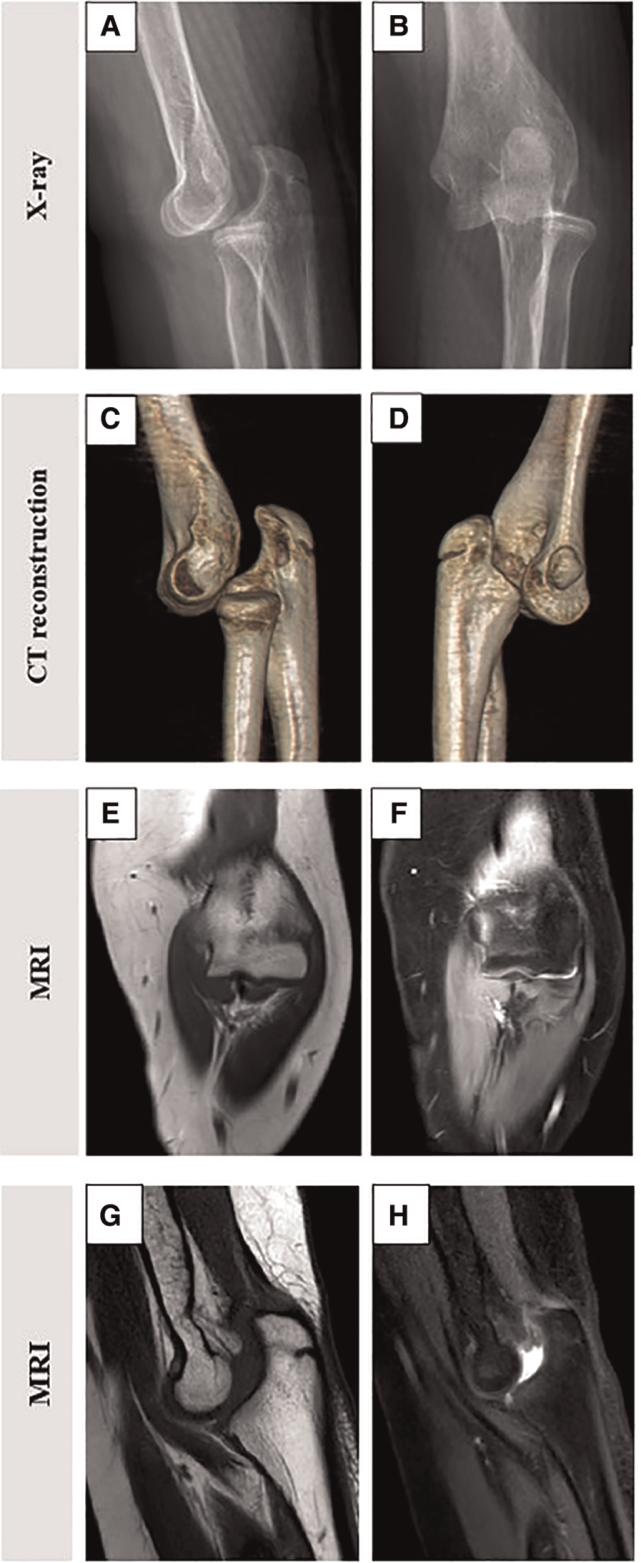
Preoperative imaging showing posterolateral dislocation of the left elbow. (A,B) Radiographs show anteriorly inferior slippage of the distal humerus without fracture; (C,D) Three-dimensional computed tomography shows a transverse defect of the coronoid process of the ulna, (E,F) The MRI of left elbow shows no injury of medial collateral ligament and lateral collateral ligament. (G,H) The MRI of left elbow shows posterolateral dislocation.
